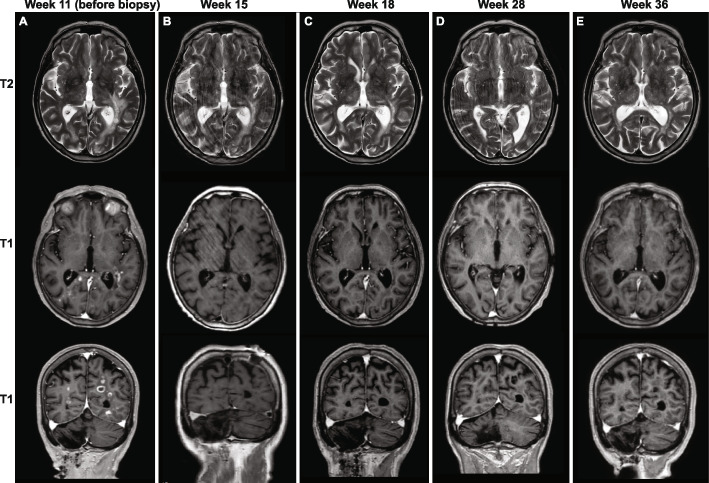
Chronological changes in brain magnetic resonance imaging (MRI). T2-flair on the top row and T1-flair on the next two rows. A 11 weeks after the symptoms started, i.e., 18 days before the biopsy, patchy T2 hyperintensities in the white matter are seen. The lesions are periventricular, mostly around the occipital- and temporal horns, with a left-sided predominance. There are also some subcortical lesions, nodular contrast enhancement in smaller lesions, and ring-like patterns in the more extensive lesions. The lesions are partly confluent with surrounding edema. B 15 weeks after the symptom started, i.e., 10 days after the biopsy, there is still some edema but almost complete regression of the contrast enhancement in the lesions. The patient was moving slightly. C 18 weeks after symptoms started, there were no or only minimal changes from the previous examination. D 28 weeks after symptoms started, there were no or only minimal changes from the previous examination. E 36 weeks after symptoms started, there were no or only minimal changes from the previous examination
